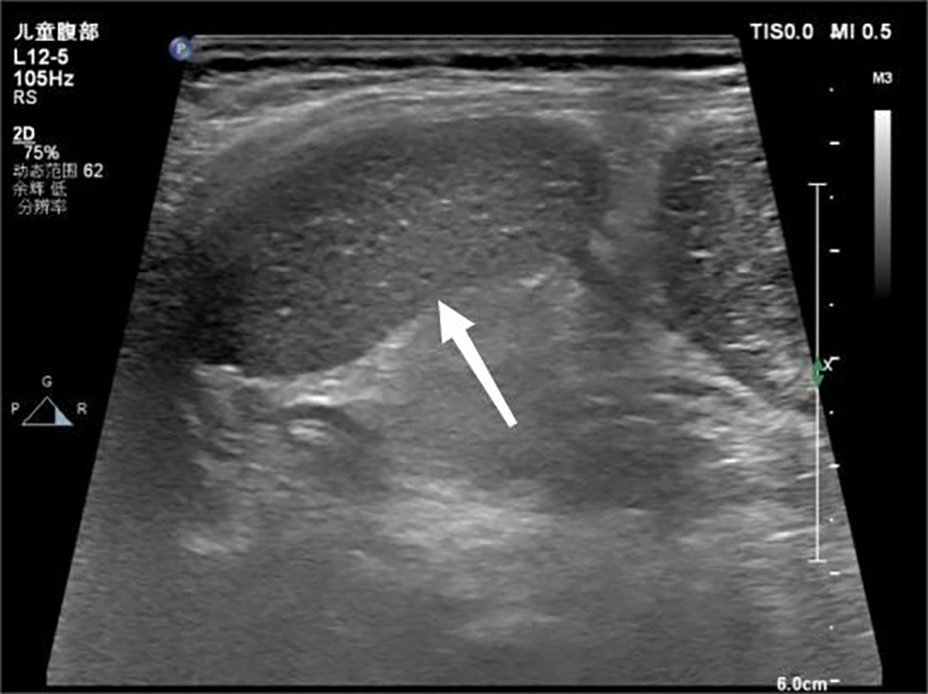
Ultrasound image showing the accessory spleen in the splenic region.
